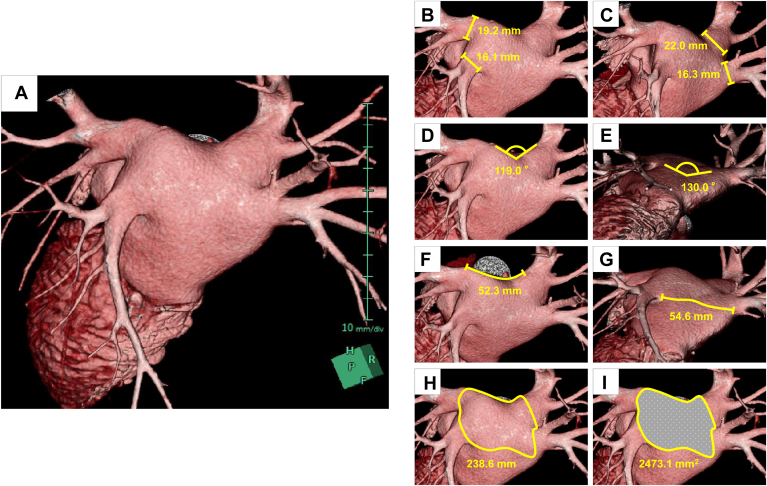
Representative preprocedural CCTA findings in a patient with PerAF who had recurrence of ATA after CB-PVI, demonstrating a long LAPC (≥199.6 mm). A: Reference axial slice. B: Ostial diameters of the LSPV and LIPV. C: Ostial diameters of the RSPV and RIPV. D: LA roof angle. E: LA bottom angle. F: LA roof line length. G: LA bottom line length. H: LAPC. I: LAPA. ATA = atrial tachyarrhythmia; CB-PVI = cryoballoon-based pulmonary vein isolation; CCTA = cardiac computed tomography angiography; LA = left atrial; LAPA = left atrial posterior wall area; LAPC = left atrial posterior wall circumference; LIPV = left inferior pulmonary vein; LSPV = left superior pulmonary vein; PerAF = persistent atrial fibrillation; RIPV = right inferior pulmonary vein; RSPV = right superior pulmonary vein.
